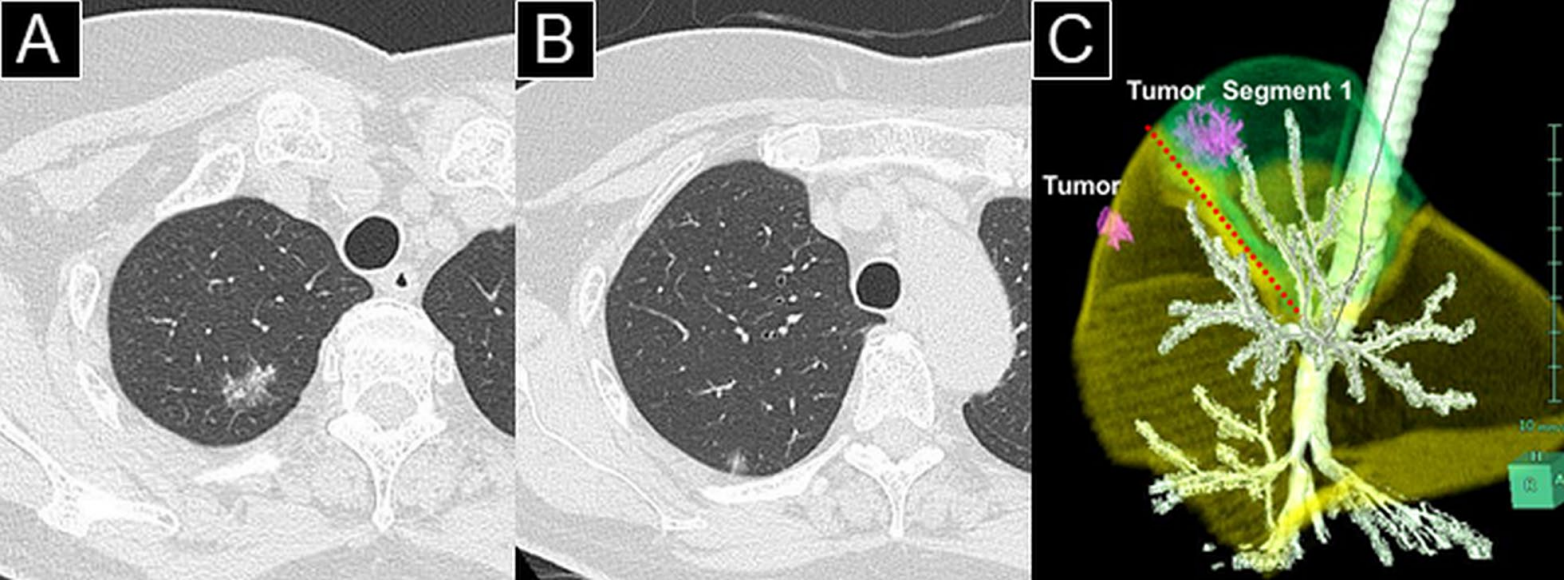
Preoperative chest computed tomography images and a three-dimensional image of the tumors. A Chest computed tomography showed a partially solid ground-glass nodule located in right segment 1. B A pure ground-glass nodule was located in right segment 2. C The tumor in segment 1 (green area) was near the boundary between segments 1 and 2 (red dotted line)
